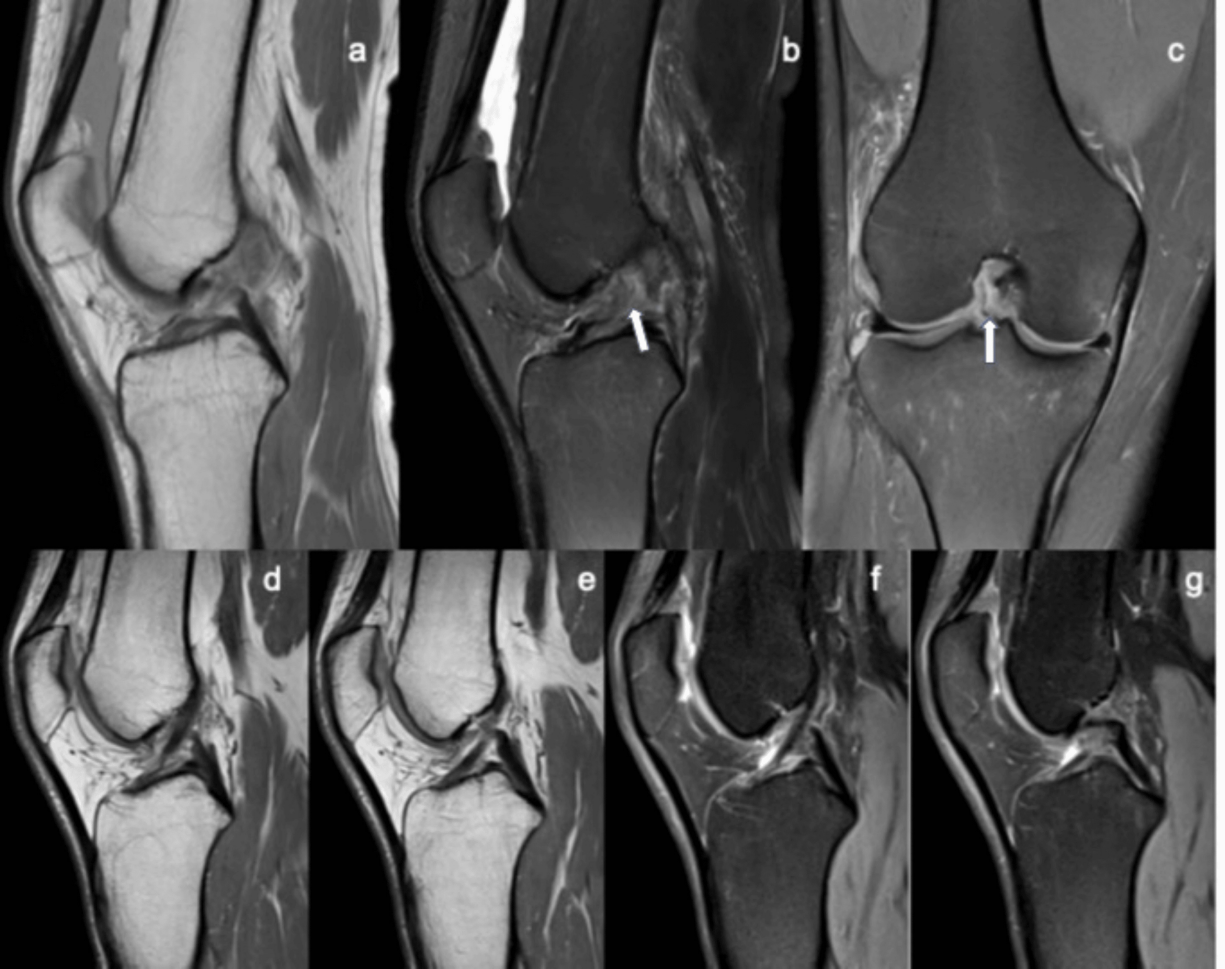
Magnetic resonance imaging 10 days after ACL injury and after 22 weeks of biological conservative treatment. Case 2: magnetic resonance imaging baseline proton density sagittal (a), proton-density fat-saturated sagittal (b), and coronal (c) at 10 days shows grade III ACL rupture (white arrows). Follow-up at 22 weeks post-PRP treatment - anterior cruciate ligament continuity and low signal are observed (repair grade 0) (d-g). PRP: platelet-rich plasma; ACL: anterior cruciate ligament
